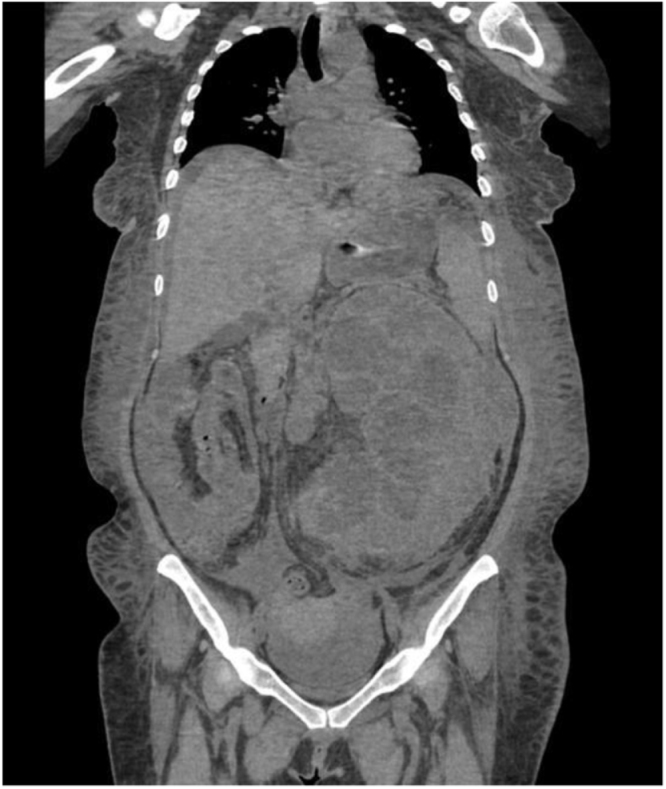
Abdominal computed tomography showed that the left kidney was significantly enlarged and presented as the “bear’s paw sign”.
